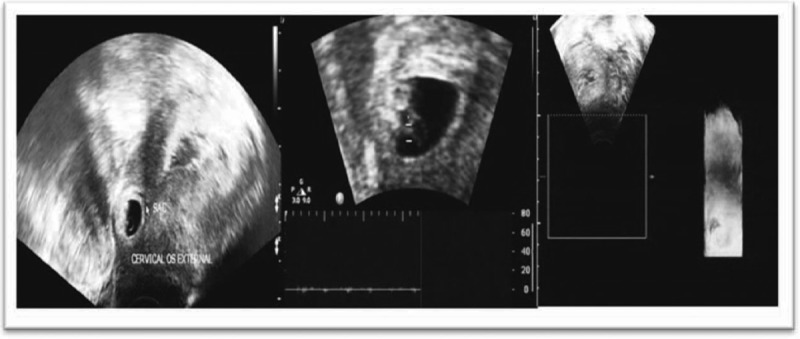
TVUS and 3D TVUS showing a cesarean scar pregnancy gestational sac with a viable fetus located in the anterior part of the isthmic portion of the uterus with diminished myometrial layer between the bladder and the sac. 3D = 3-dimensional; TVUS = transvaginal ultrasound.
